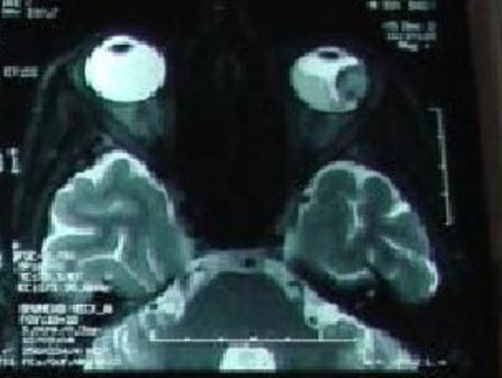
A left lateral choroidal mass with secondary retinal detachment on magnetic resonance imaging.
